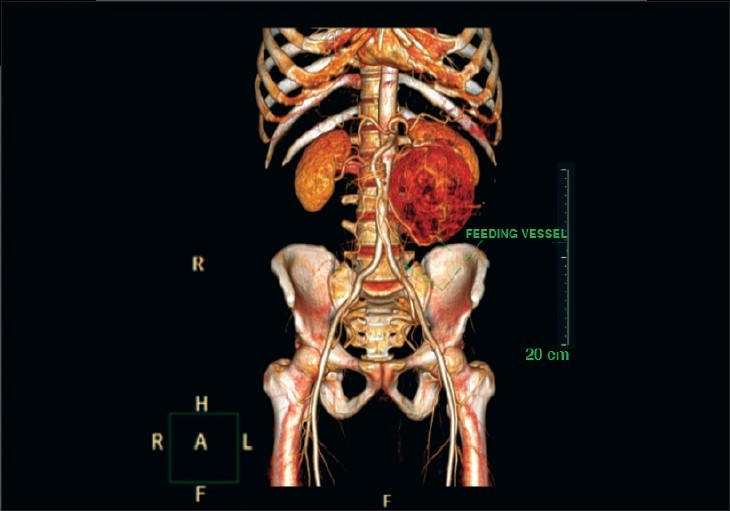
Computed tomography angiogram showing a tumor situated in front of the left kidney. The left kidney and adrenals are normal
